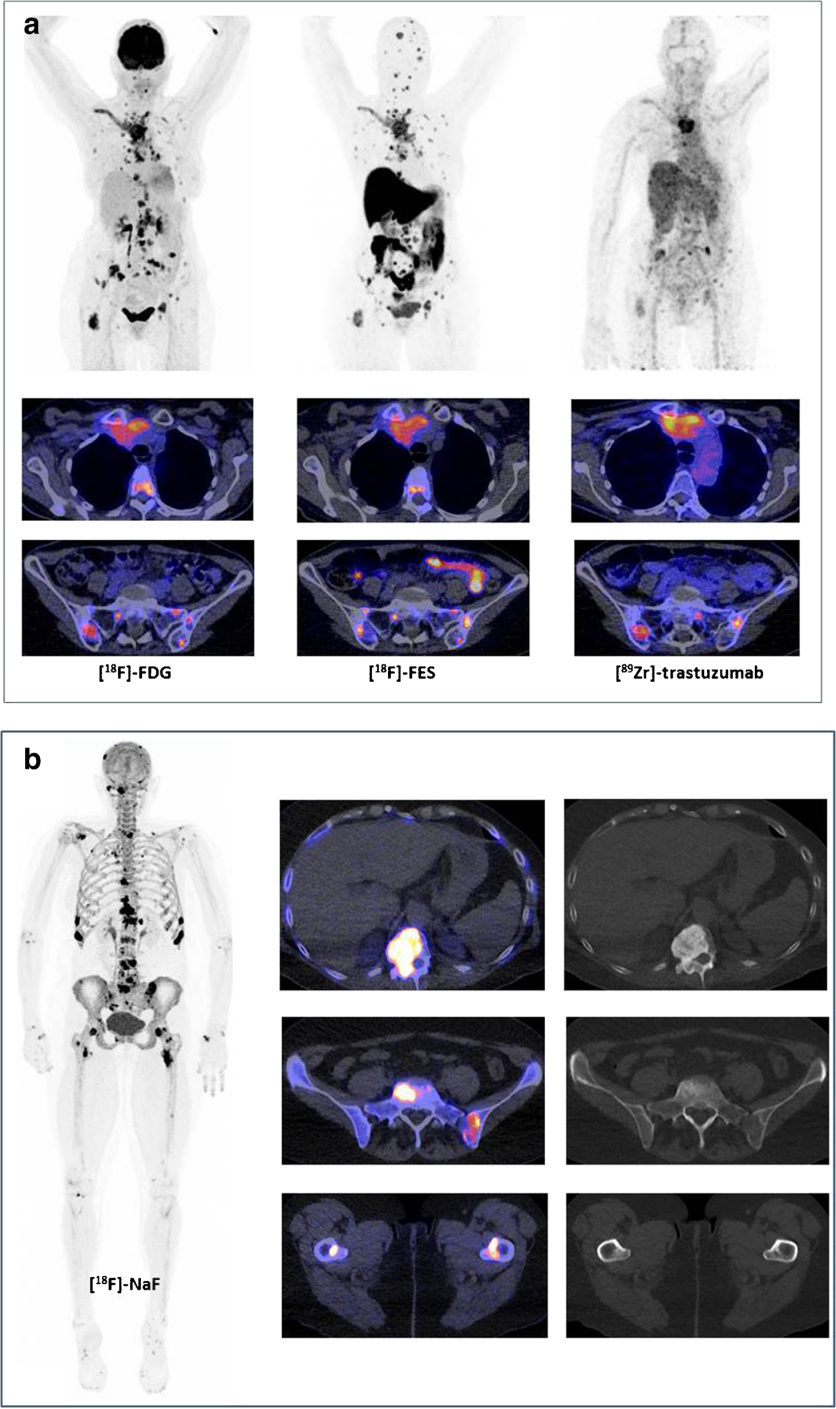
Upper image: three PET scans ([^18^F]-FDG-PET, [^18^F]-FES-PET, and [^89^Zr]-trastuzumab-PET) in the same patient showing mediastinal and hilar lymph node metastases, as well as intrapulmonary lesions visible on both [^18^F]-FDG-PET and [^18^F]-FES-PET, but not on [^89^Zr]-trastuzumab-PET. The large mediastinal mass (first row of transversal fused images) was visible on all three imaging modalities. Bone metastases (second row of transversal fused images) were clearly visualized on [^18^F]-FES-PET, for example, skull lesions, and to a lesser extent on [^18^F]-FDG-PET and [^89^Zr]-trastuzumab-PET. Lower image: [^18^F]-NaF-PET in another patient showing bone metastases in the skull, vertebrae, costae, pelvis, and proximal femora. The increased uptake in the joint was related to degeneration
